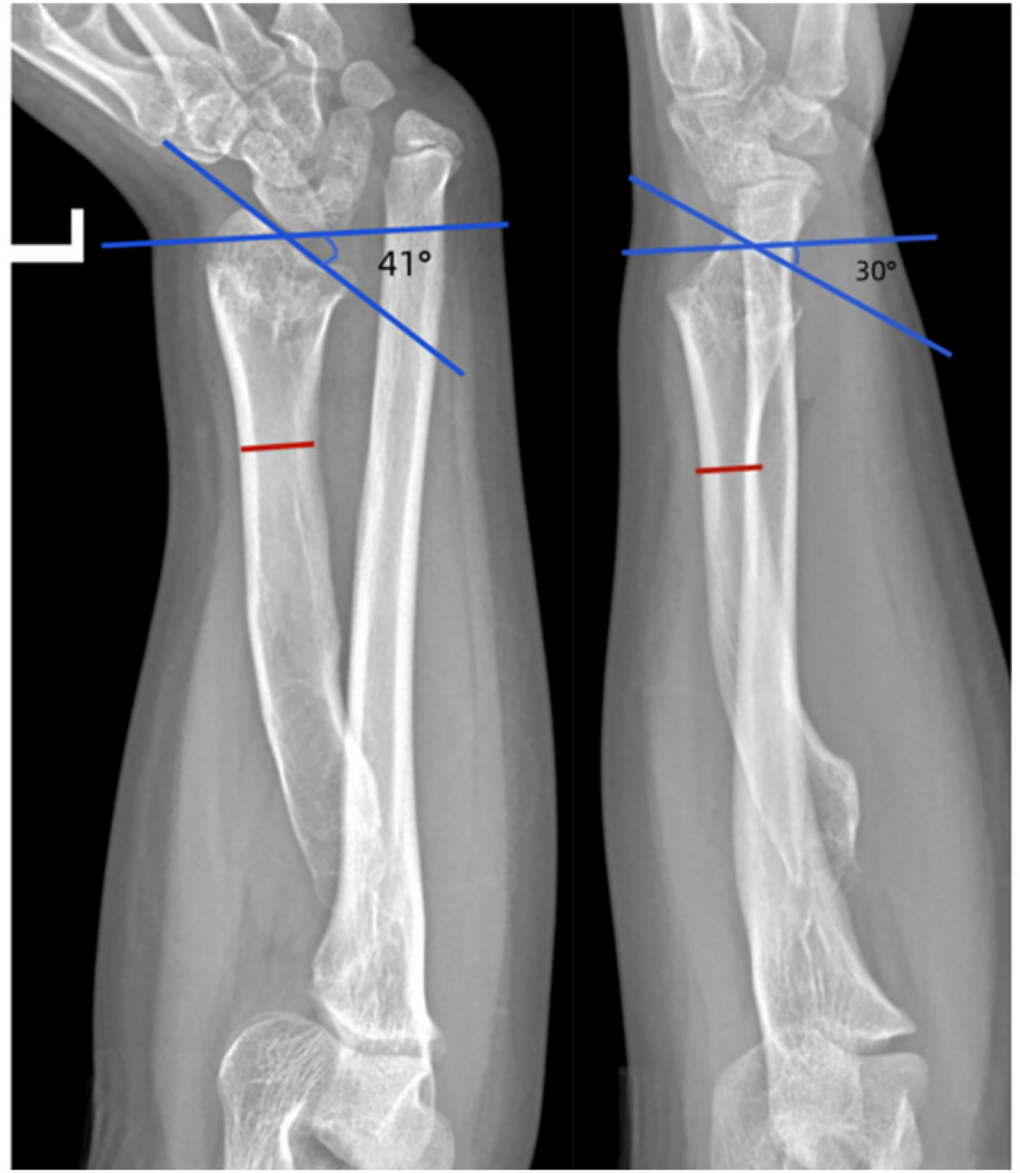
Anteroposterior and lateral radiographs demonstrating Madelung deformity. The blue-marked angles indicate the ulnar inclination and the volar tilt. The red line denotes the osteotomy plane.
